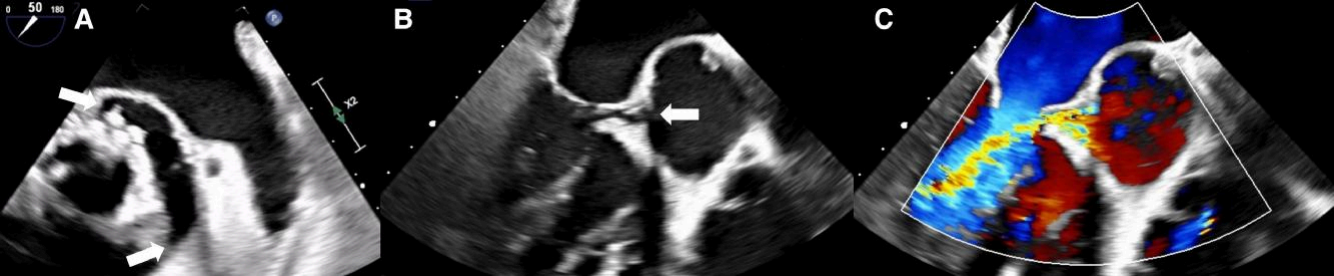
(*A*) Severe paravalvular detachment of about 120° of the circumference of the bioprosthetic aortic valve, short axis. (*B*) Valve dehiscence, three-chamber view, long axis. (*C*) Severe paravalvular insufficiency, colour Doppler, three-chamber view, long axis.
